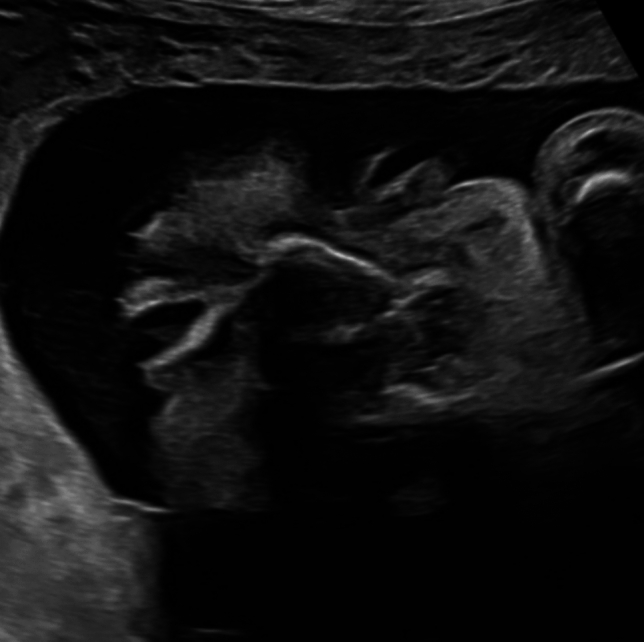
2D ultrasound in the frontal plane failed to demonstrate the closed eyelids
